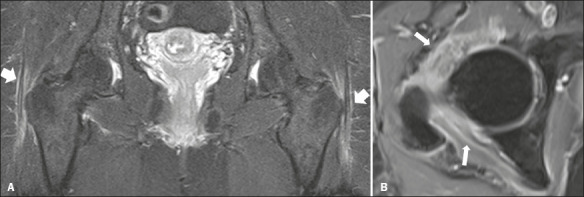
MRI of the hips in a 72-year-old female with PMR. A: Coronal T2wFS image
